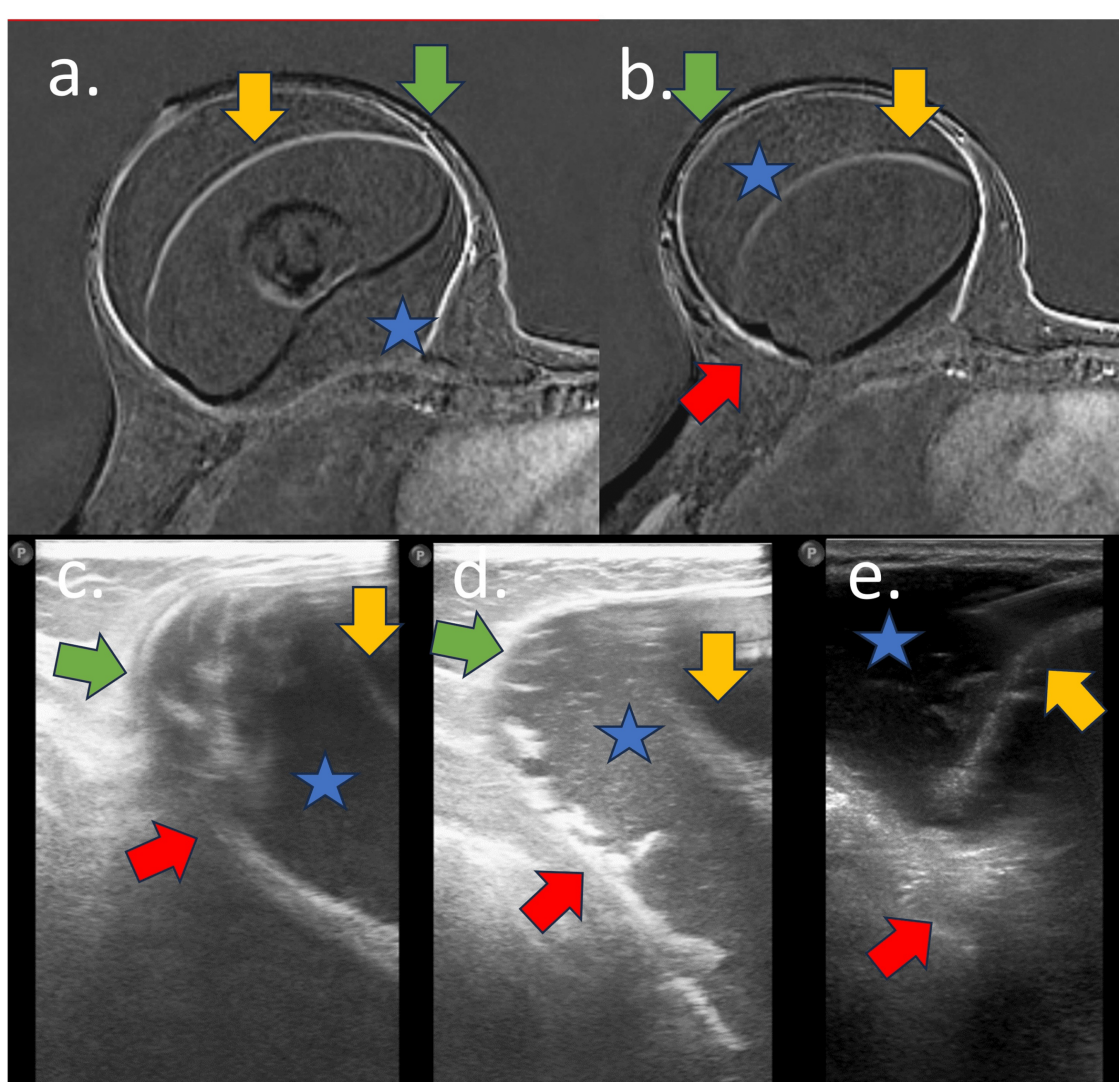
Early complications of acellular dermal matrix (ADM): USG and MRI Same patient as Figure 8. (a and b) Axial post-contrast sequence and (c, d, and e) B-mode USG. Voluminous intracapsular collection (blue star) with fibrous capsule irregularity (green arrow), implant surface (yellow arrow), intracapsular heterogeneous tissue compatible with ADM (blue arrow), and intracapsular vegetation (red arrow)
